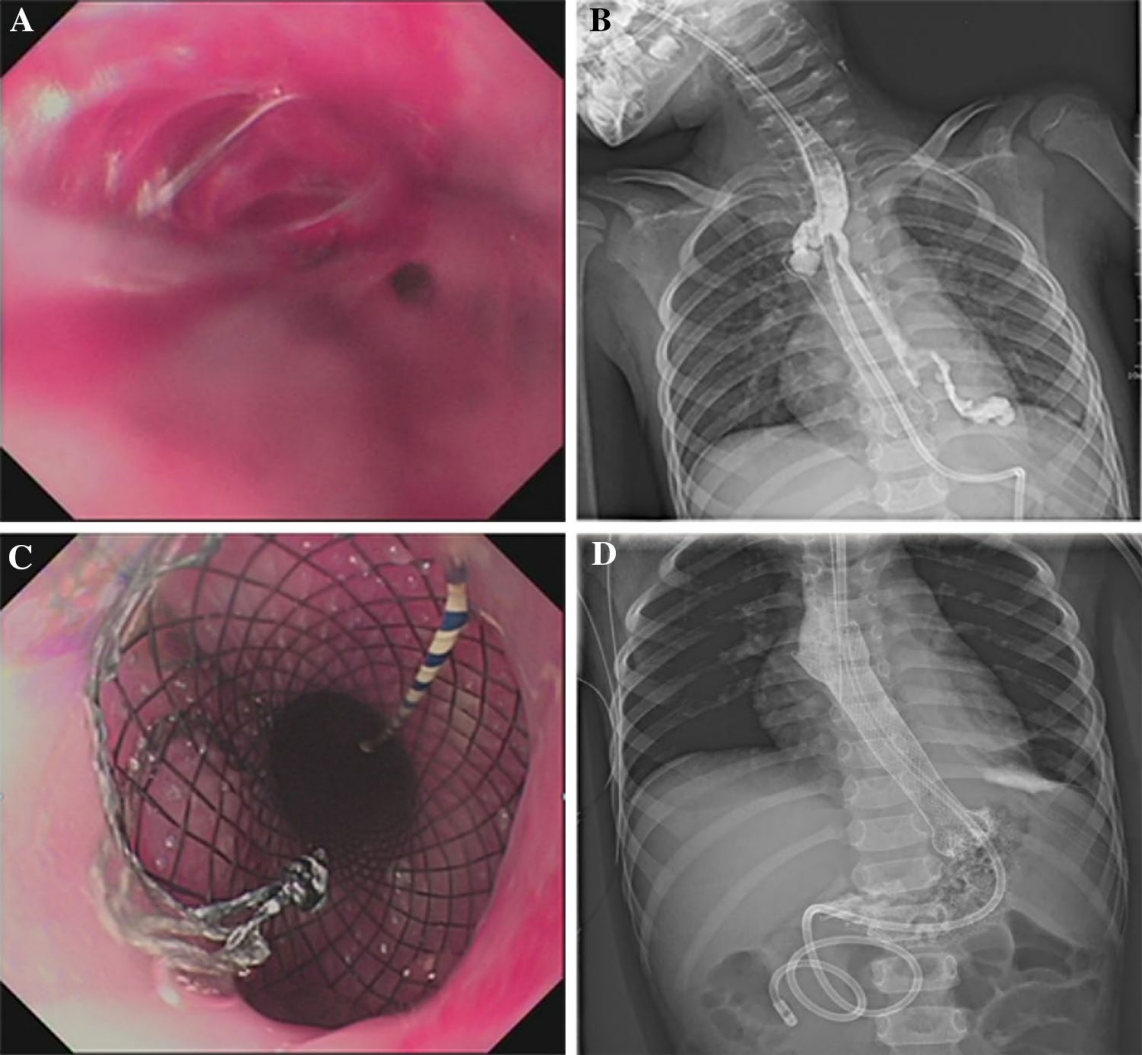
Stent placement in a patient with an EBD-induced esophageal perforation (case 14). (A) Esophageal perforation secondary to endoscopic balloon dilatation, the upper oral mucosa of the stricture was torn and bleeding; (B) Stent malapposition occurred 5 days after the first stent implantation; upper gastrointestinal tract radiography revealed that contrast medium infiltrated the mediastinum; (C) The original esophageal stent was replaced by a redesigned stent with larger upper bell mouth; (D) After the second esophageal stent was placed, upper gastrointestinal tract radiography revealed that the fistula was plugged, without contrast medium extravasating.
